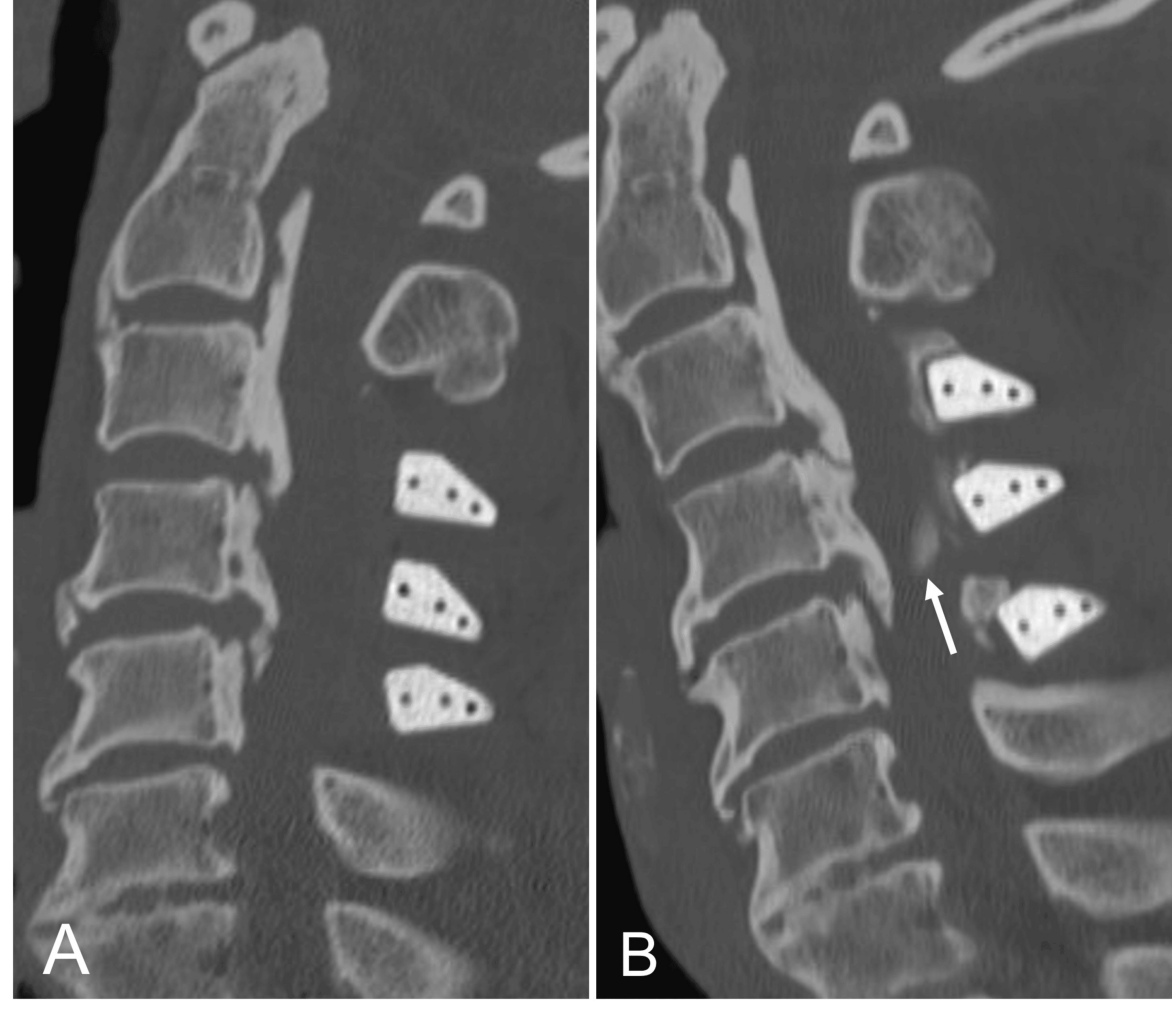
CT images of the cervical spine in the case that required reoperation Sagittal view of the cervical spine CT images immediately (A) and 102 months (B) after surgery of the OPLL patient who required reoperation showing osteophyte-like new bone formation resulting in spinal canal stenosis at the C4/5 level (white arrow). No obvious bone bridging between spacers was observed. CT: computed tomography, OPLL: ossification of the posterior longitudinal ligament
